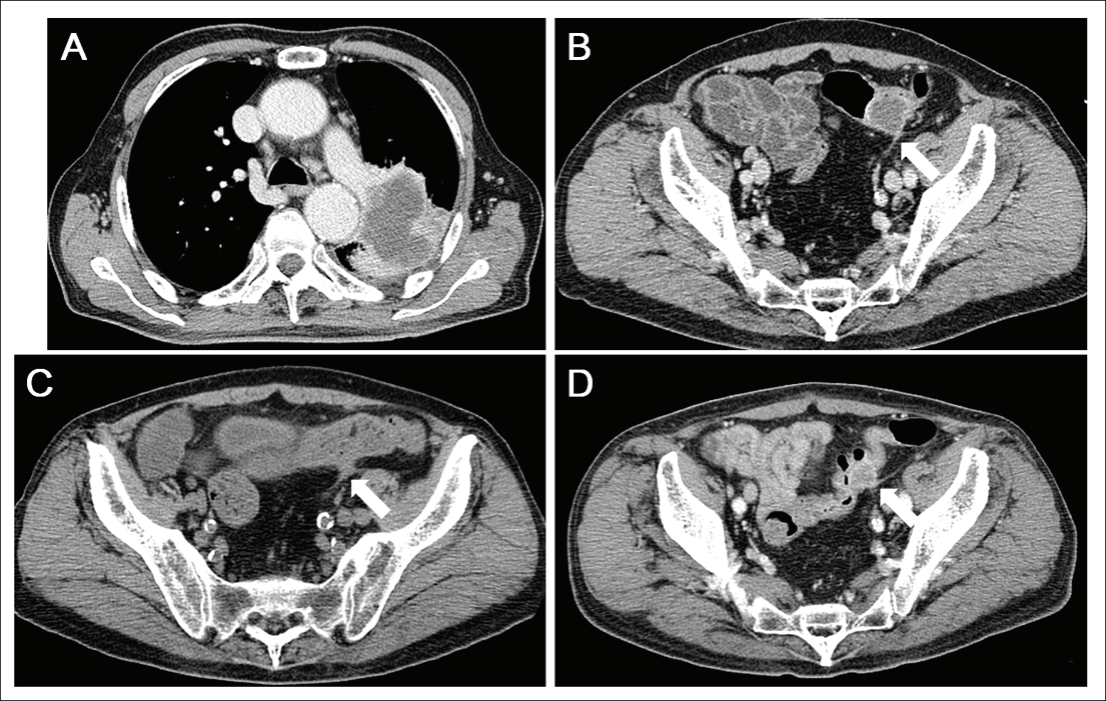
CT scanning images. (a) Lung cancer in the left-upper lobe measuring 7.8 cm in diameter. The tumour invaded both the pleura and the outer layer of the descending aorta. Central necrosis was shown. (b) A metastatic tumour in the sigmoid colon. (c) During chemotherapy, the tumour in the sigmoid colon decreased in size, whereas the primary lung tumour and other metastatic tumours were unchanged. (d) Four months after the diagnosis of lung cancer, the sigmoid colon tumour was enlarged again.
